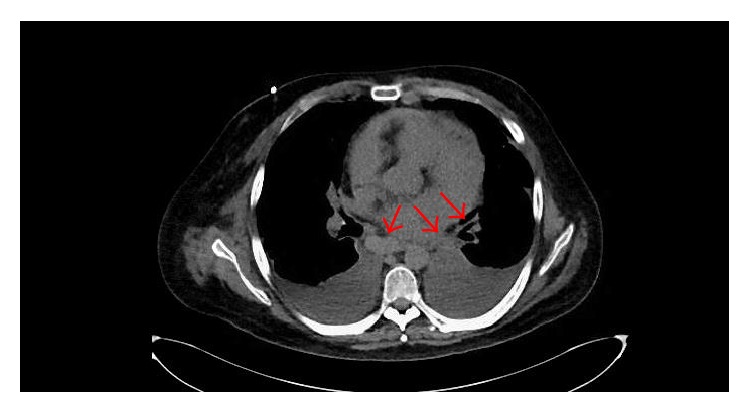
Chest CT with circumferential pericardial and nodular soft tissue densities posterior to the heart as denoted by red arrows.
